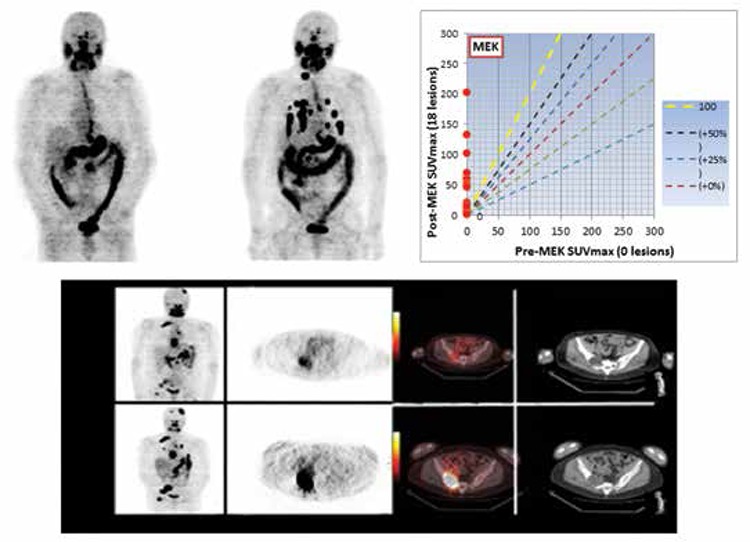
A thyroid cancer patient with widely metastatic neck nodes, pulmonary nodules, mediastinum, and osseous sites. The upper row is the baseline study before seluemetanib treatment (left), and the post four week MEK treatment image showing a number of pulmonary lesions now taking up the radioactive iodine. The graph (top, right) compares the standardized uptake values (SUV) before (abscissa) and after (ordinate) treating in quantitative terms. No lesions were detectable in terms of 124I prior to treatment and subsequently, the uptake that developed was seen. There is a different patient showing baseline (upper row) and the re-induction effects of treatment on radioiodine uptake (bottom row). There are new lesions in the lungs and the osseous lesions have greater uptake (left panel coronal image baseline, above and post-treatment below); cross-sectional imaging 124I imaged on positron emission tomography, baseline (above) and post-treatment (below). There is marked increase in the intensity of uptake in the R. pelvis, and the relationship to computed tomography findings is dramatically displayed in the fusion positron emission tomography/computed tomography, which shows a space occupying lesion in the R. ileum, now with therapeutic levels of uptake. As part of the selumetinib pilot trial, we have been able to document dose-response relationships between 124I lesional dosimetry and subsequent structural disease response as determined by Response Evaluation Criteria in Solid Tumors criteria in eight differentiated thyroid cancer patients (1).
